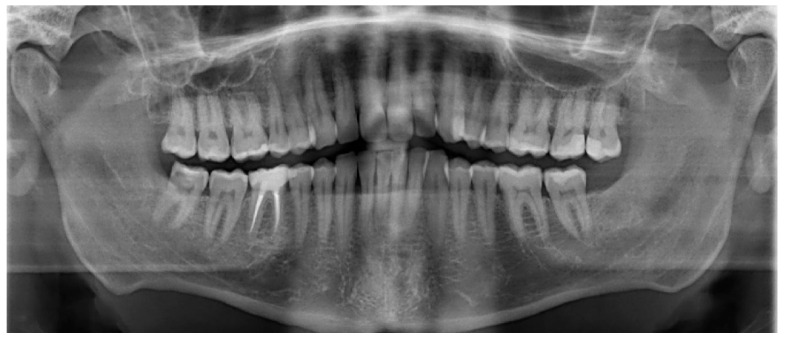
Postoperative panoramic radiograph showing normal bilateral temporomandibular joint (TMJ) articulation. No signs of odontogenic infection, recurrence, or structural abnormalities are present.
